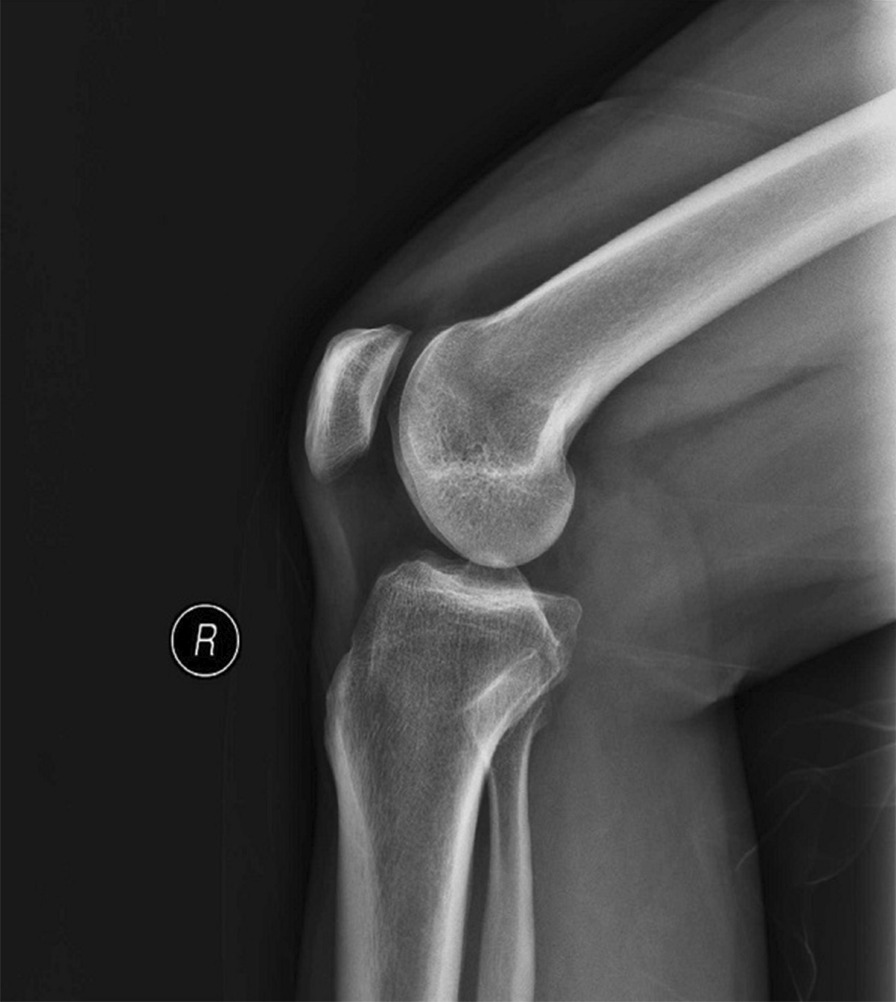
Radiograph of lateral knee joint captured in optimized
